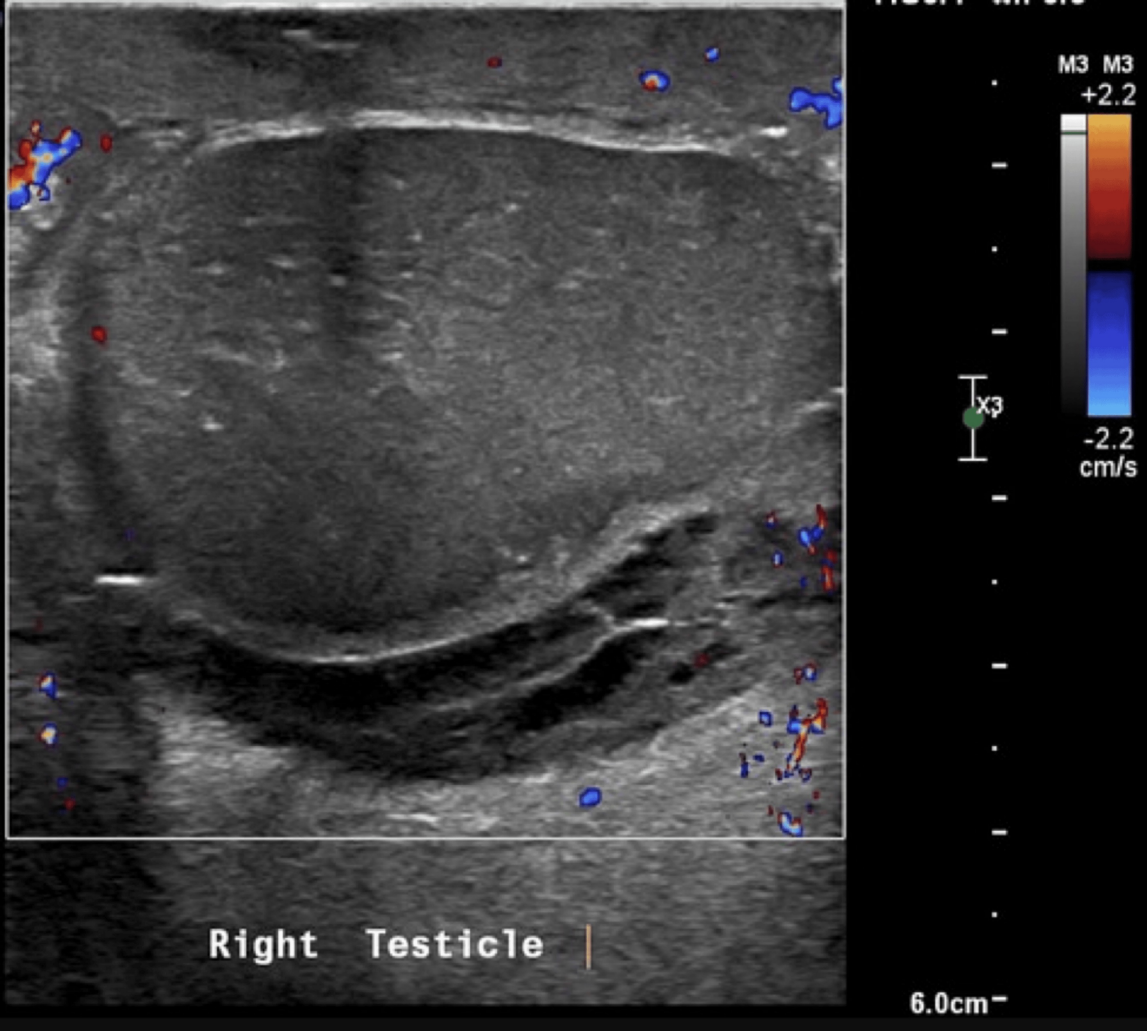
Color Doppler ultrasound of right testicle showing no vascularity
